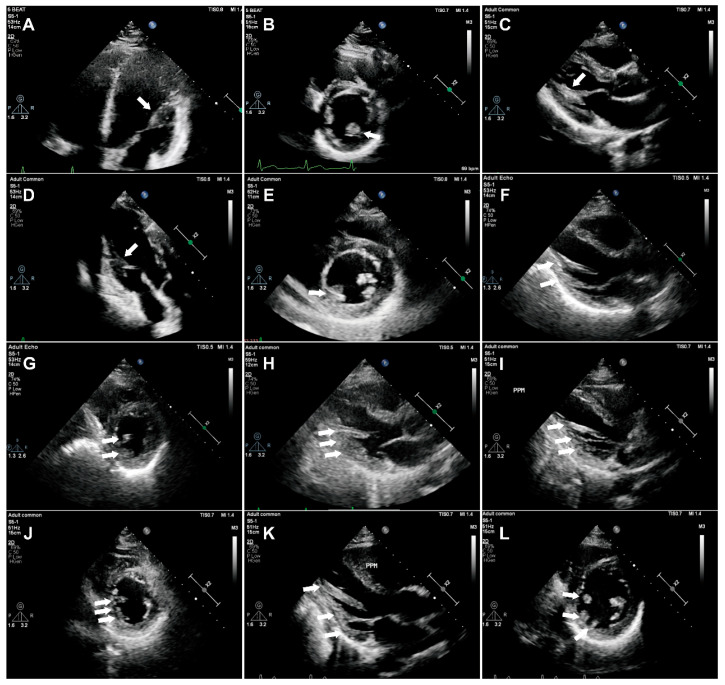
Echocardiographic images of left ventricular papillary muscle morphological classifications. (A,B) Long-axis and short-axis views of anterolateral papillary muscle (APM) IA type; (C) long-axis view of posteromedial papillary muscle (PPM) IB type; (D,E) long-axis and short-axis views of PPM IIA type; (F,G), long-axis and short-axis views of PPM IIB type; (H) long-axis view of PPM IIIA type; (I,J) long-axis and short-axis views of PPM IIIB type; (K,L) long-axis and short-axis views of PPM IIIC type.
